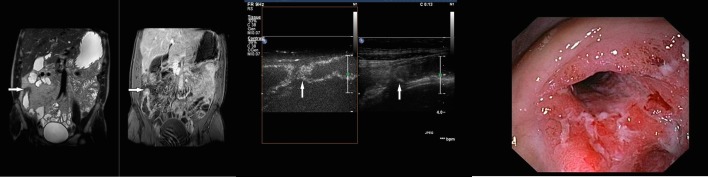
Thirty-one-year-old female patient with Crohn’s disease and stenosis of the ileocolic anastomosis after ileocecal resection. A: Coronal MR Enterography images (T2-weighted single-shot turbo spin-echo on the left image; T1-weighted fat saturated, spectrally attenuated inversion recovery after Gadoteric acid administration, on the right image) of a patient with stenosis (white arrow) and increased enhancement of the ileocolic anastomosis (white arrow). B: Longitudinal Contrast enhanced ultrasound (Gray scale ultrasound image, on the right side; Contrast enhanced ultrasound image, on the left side), of the same patient with a short stenosis of the ileocolic anastomosis (white arrow) and increased enhancement after Sonovue administration (white arrow). C: Endoscopic image of the same patient showing an ulcerative stenosis of the neoterminal ileum.
